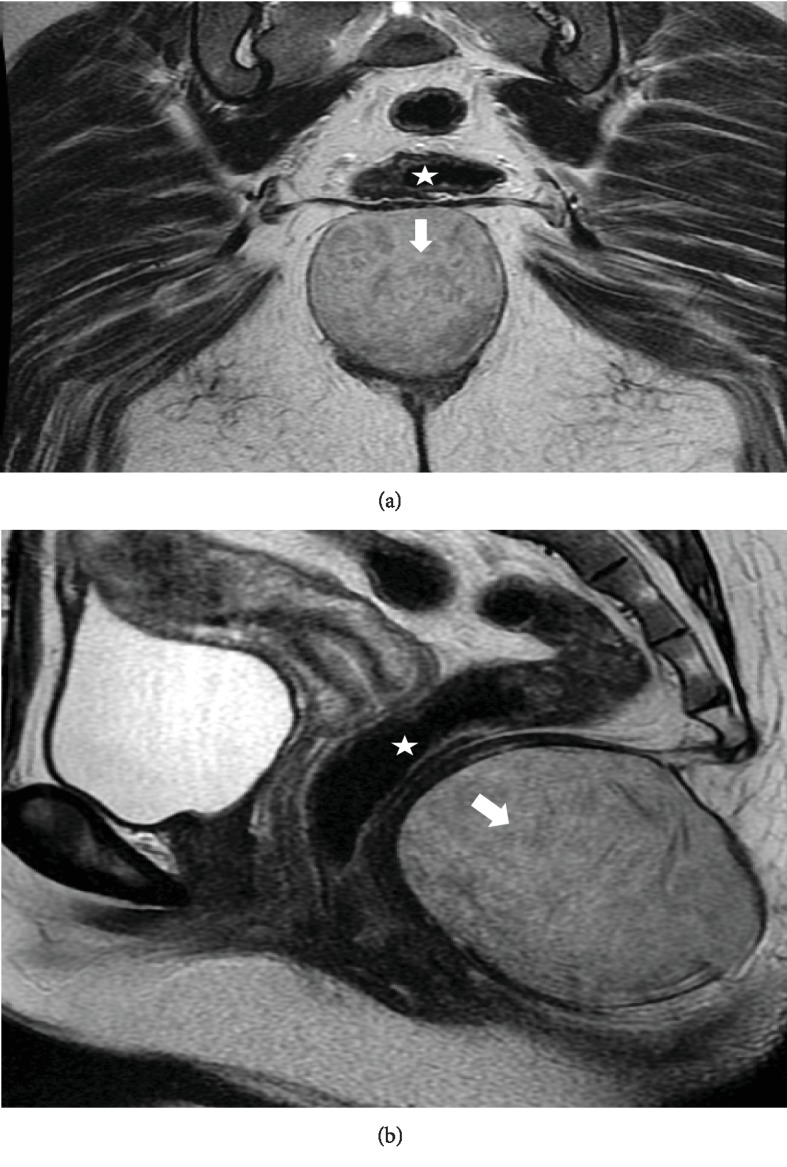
Perineal epidermoid cyst in a 30-year-old woman. Mass-oriented magnetic resonance imaging showing (a) coronal T2-weighted images (T2WI), and (b) sagittal T2WI. The lesion is indicated with a white arrow. T2WI demonstrated a well-circumscribed homogeneous structure located in the middle of the perianal region and posterior to the rectum (star); the lesion has an intermediate to high signal intensity, suggesting a fluid containing the cystic lesion. In unshown images, the lesion is hypointense relative to surrounding fat tissue on T1WI with no enhancement after gadolinium administration, only slight peripheral rim enhancement.
